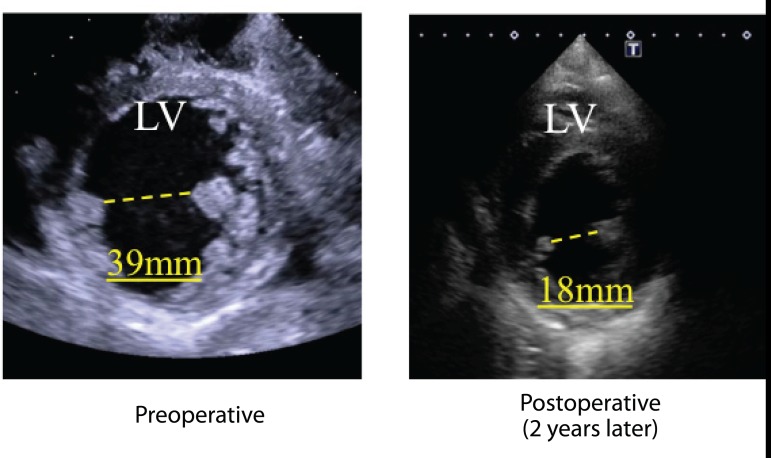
Pre and postoperative transthoracic echocardiography. In the short axis view, the anterior and posterior papillary muscle distance (interpapillary muscle distance) was 39 mm in preoperative echocardiography. Two years later, interpapillary muscle distance was 18 mm.
